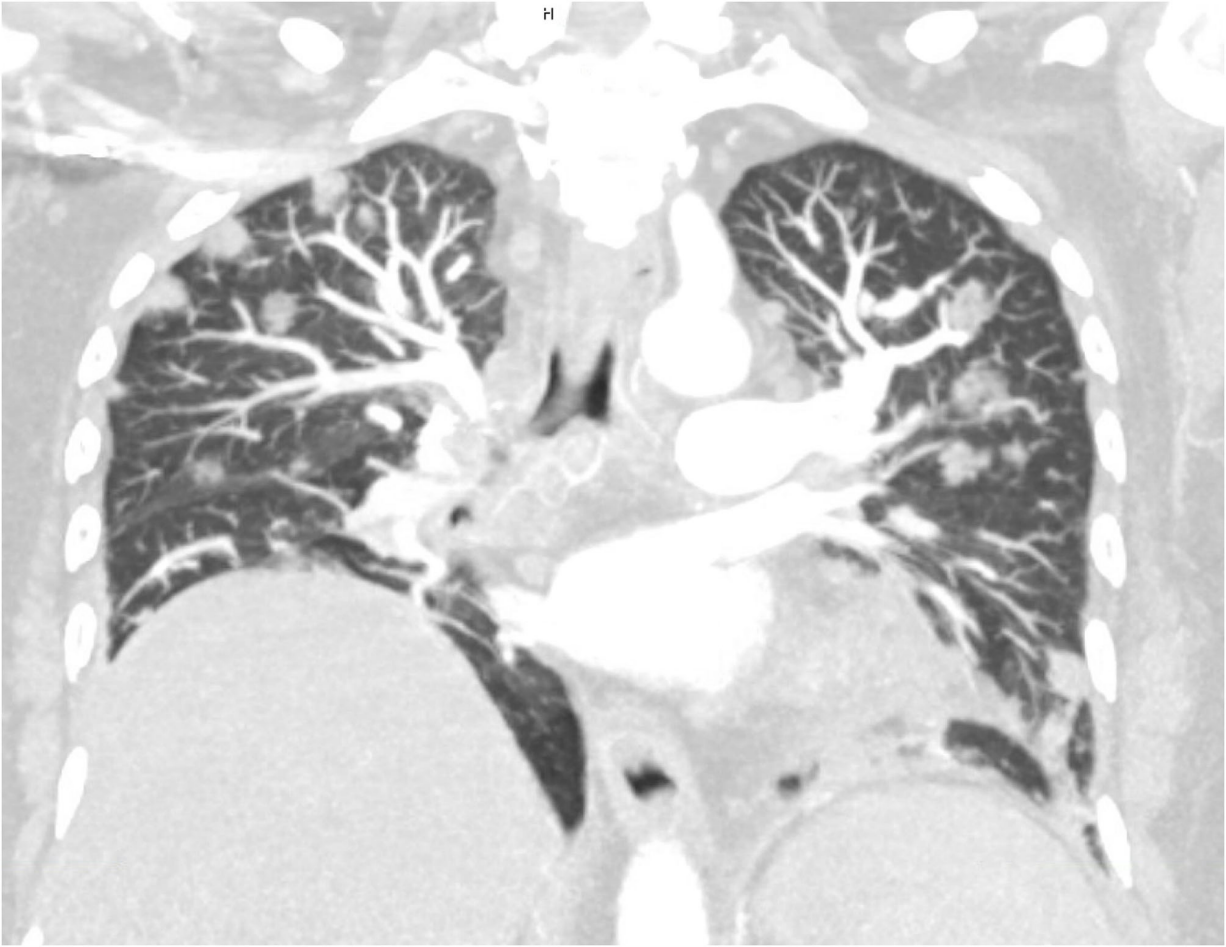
Maximal intensity projection (MIP) of multi-planar reconstruction computed tomographic angiography at admission day. Multiple septic embolic lesions appearing as nodules are present predominantly in the subpleural as well as peribronchovascular area, while close topographic proximity to the branching pulmonary arteries is noted.
